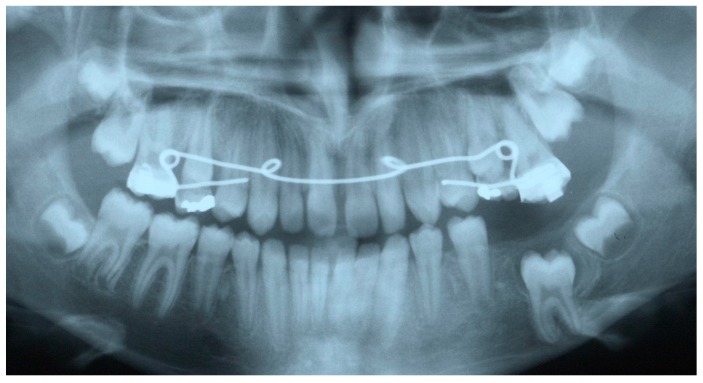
Panoramic radiograph after the extraction of the mandibular deciduous molars with the Quad-helix appliance.
